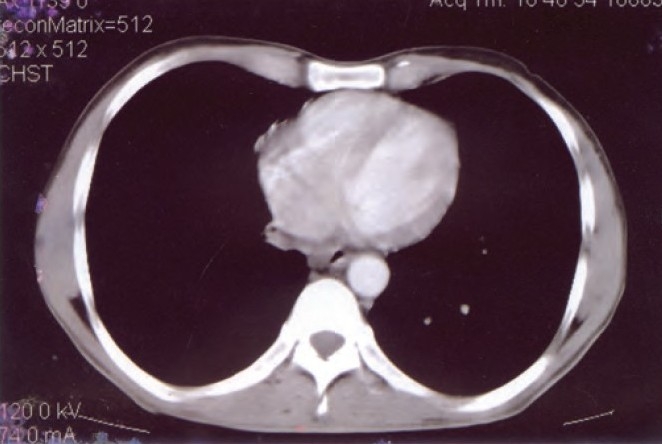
CT Scan of the thorax showing no abnormality in the lung parenchyma and no mediastinal lymphadenopathy.
